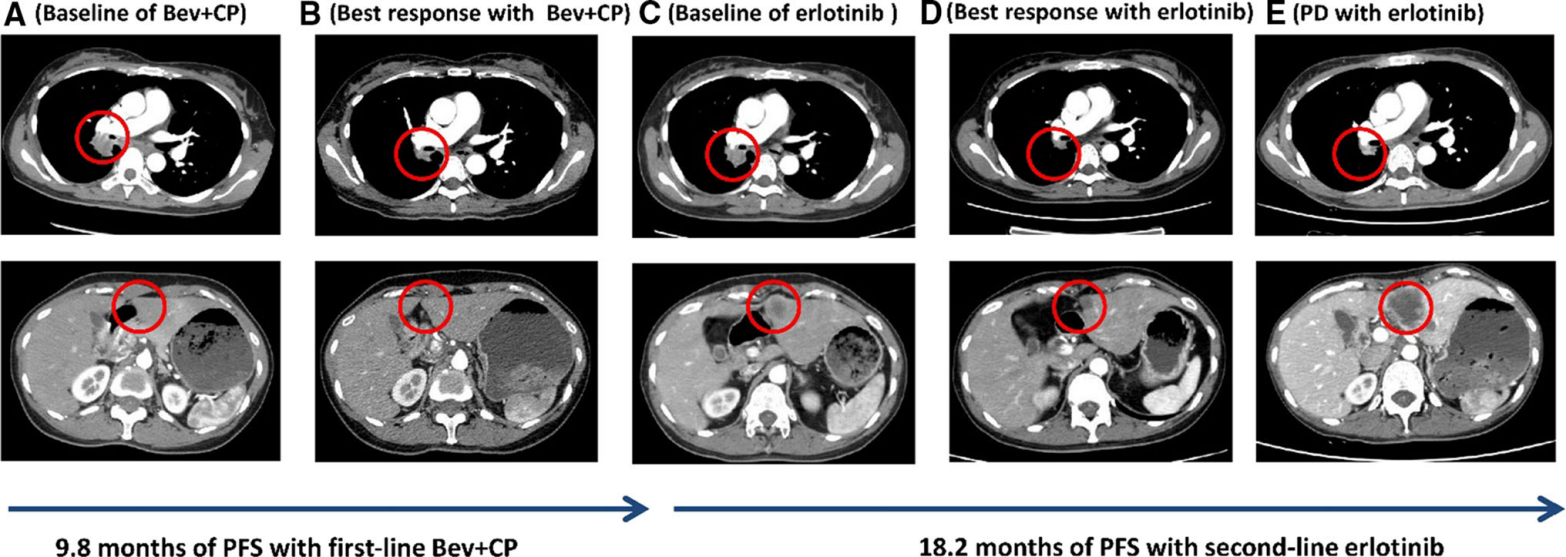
Computed tomography (CT) scans of one of our patients treated with first-line CP regimen plus bevacizumab followed by second-line EGFR-TKIs. a Chest CT scans before CP plus bevacizumab. b Partial response after 4 months with CP plus bevacizumab. c Disease progression after 9.8 months of CP plus bevacizumab treatment. d Partial response after 2 months with eroltinib. e Disease progression after 18.2 months of erlotinib treatment
